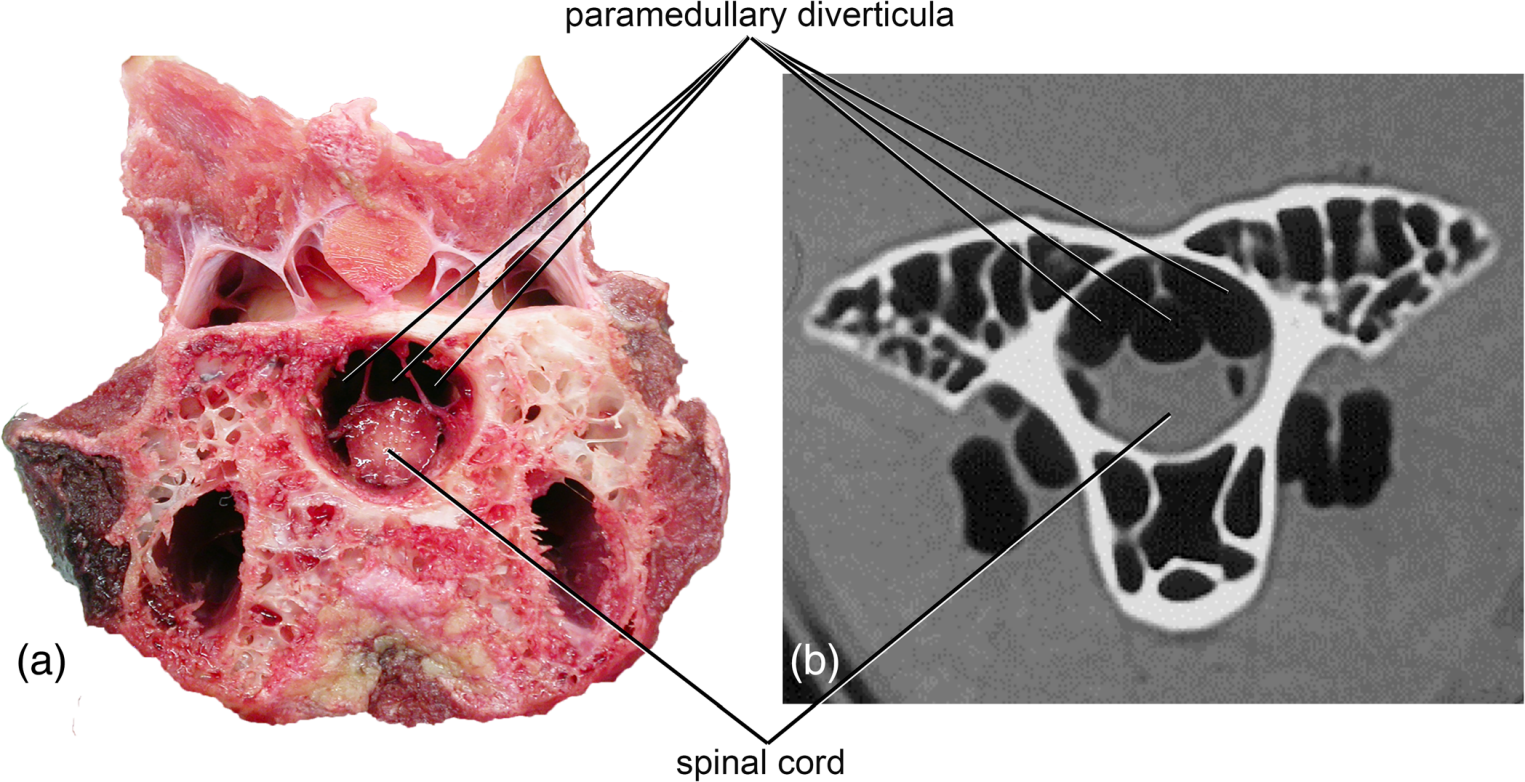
Paramedullary diverticula as seen a dissection and a CT scan. (a) Fresh dissection of an ostrich neck. (b) CT scan of an ostrich neck. In both visualizations, paramedullary diverticula are clearly visible as dark air spaces adjacent to the soft tissue of the spinal cord, contained within the hard bony tissue of the vertebral canal
